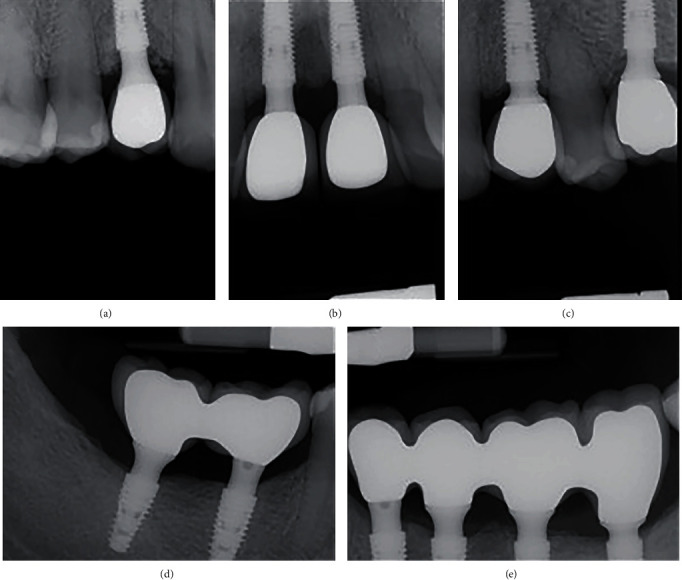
Periapical X-rays of maxillary (a)–(c) and mandibular (e, e) implants at the 24 months follow-up visit.
